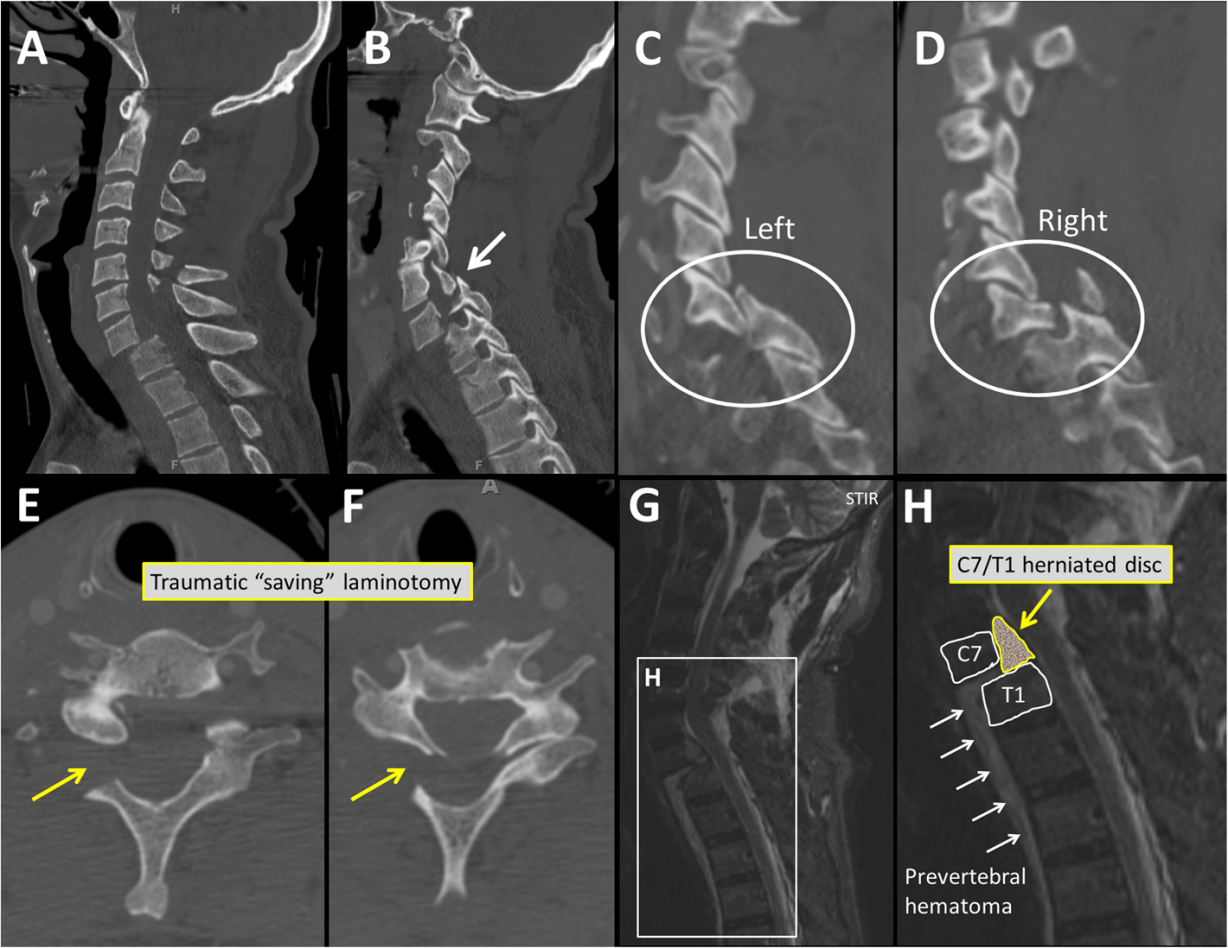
Initial diagnostic workup of the cervical spine injury with CT (panels a-f) and MRI (panels G,H). The sagittal views in panels a-d demonstrate the fracture dislocation with the left sided perched facet (panel c, and arrow in panel b) and the right sided facet fracture-dislocation (panel d). The posterior traumatic laminotomy is shown in axial CT images of C6 (panel e) and C7 (panel f). Panels g and h are sagittal STIR-weighed MRI images of the cervical spine, demonstrating the extruded C7/T1 disc within the spinal canal (panel h is a magnification of panel g
